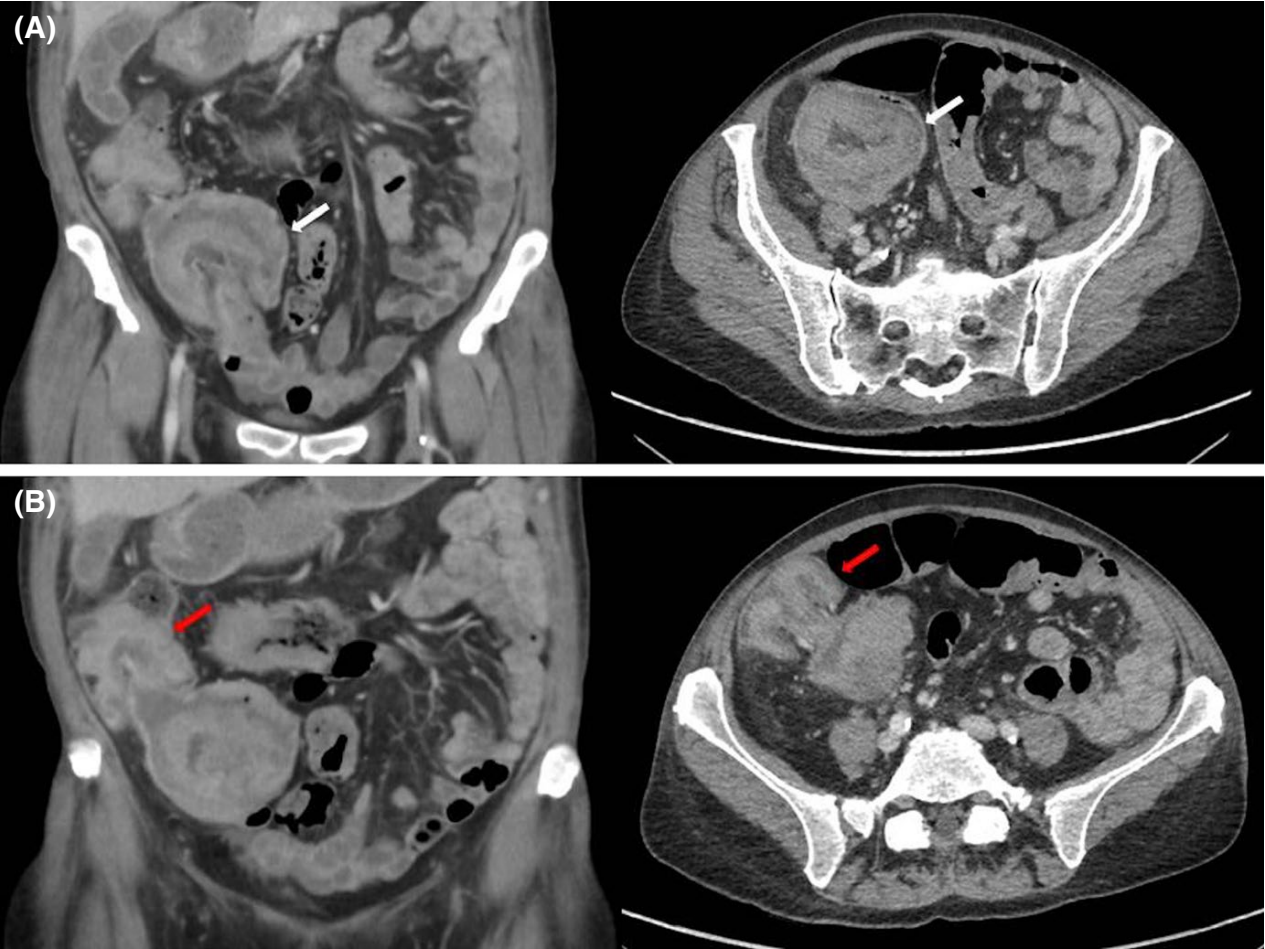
Coronal and axial planes of the computed tomography abdominal scan demonstrating a caeco‐colic (Panel A: white arrows) and a simultaneous colo‐colic intussusception (Panel B: red arrows) related to bowel metastasis of a non‐small cell lung cancer
